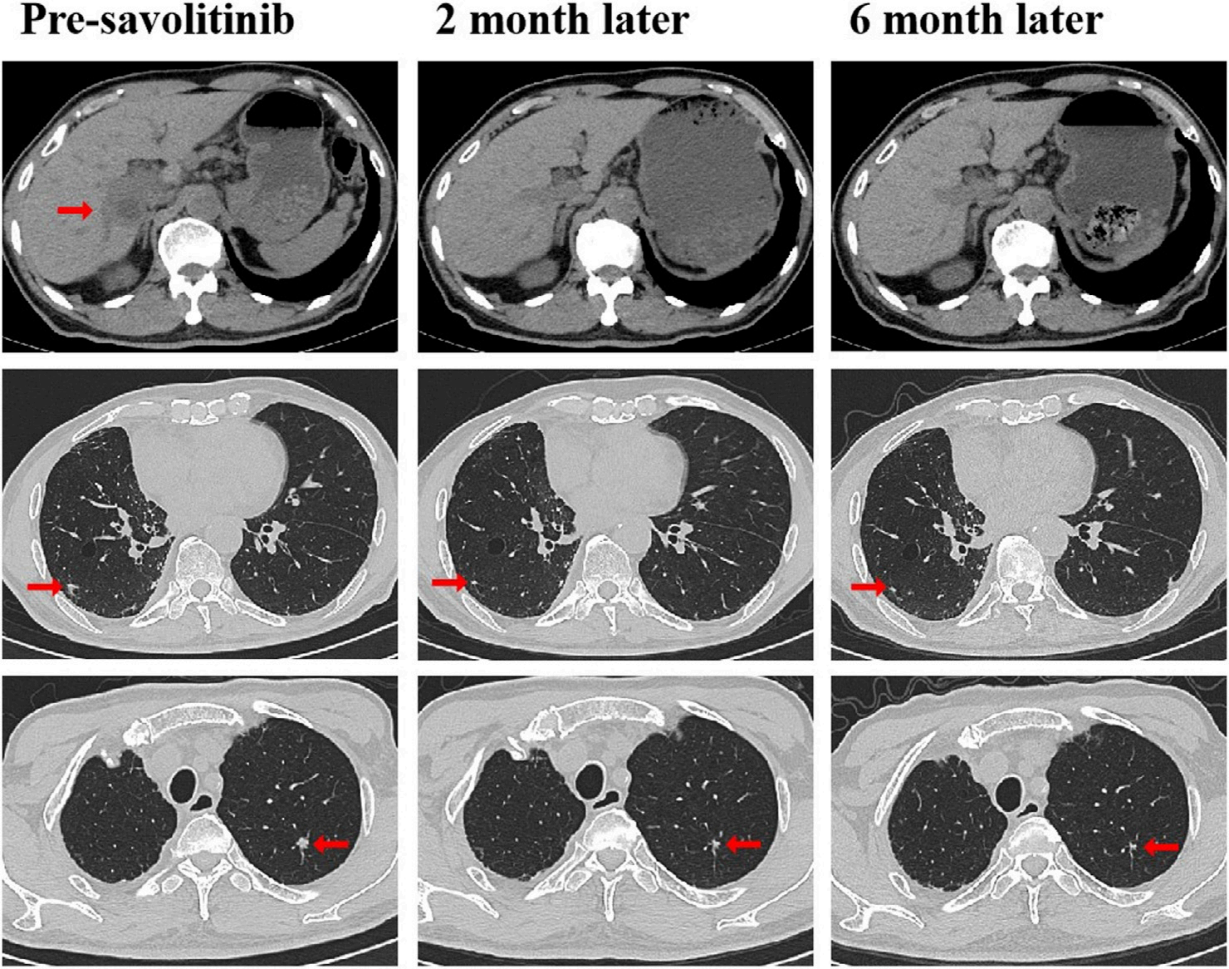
CT scans before and after savolitinib treatment. CT scans at pre-savolitinib showed the presence of liver metastasis and bilateral lung metastases. CT scans after treatment with savolitinib showed partial response in bilateral lung lesions and complete response in liver metastasis. CT, computed tomography.
